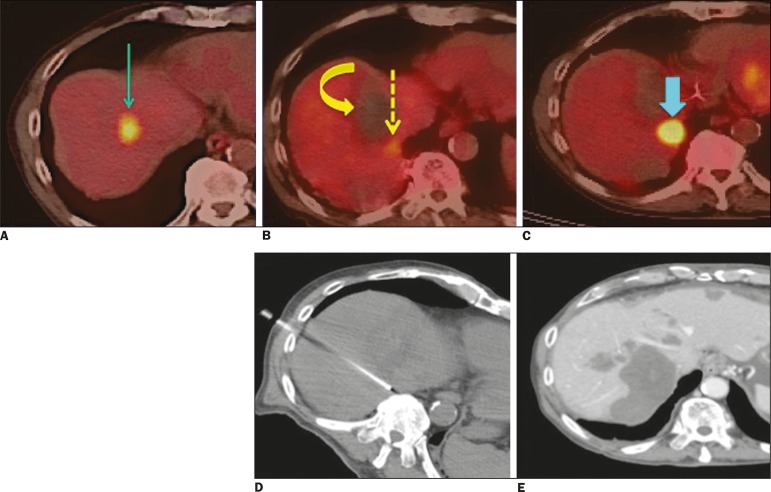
A: Baseline ^18^F-FDG PET/CT image of a liver
